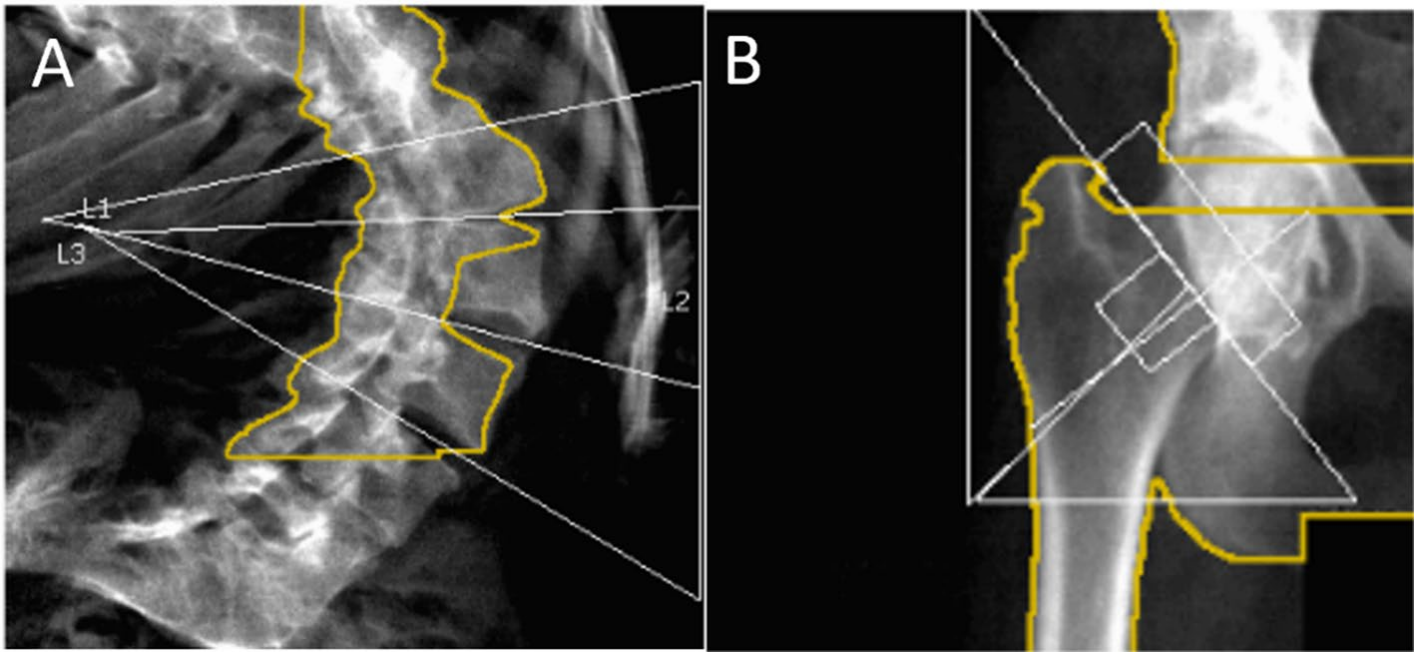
Arthrogryposis multiplex congenita and difficulties in measuring bone mineral density with dual-energy X-ray absorptiometry. The dual-energy X-ray absorptiometry results were uninterpretable for some arthrogryposis patients due to severe scoliosis (A) or hip joint contracture with mechanical arthropathy (B). These data were excluded from the analysis.
